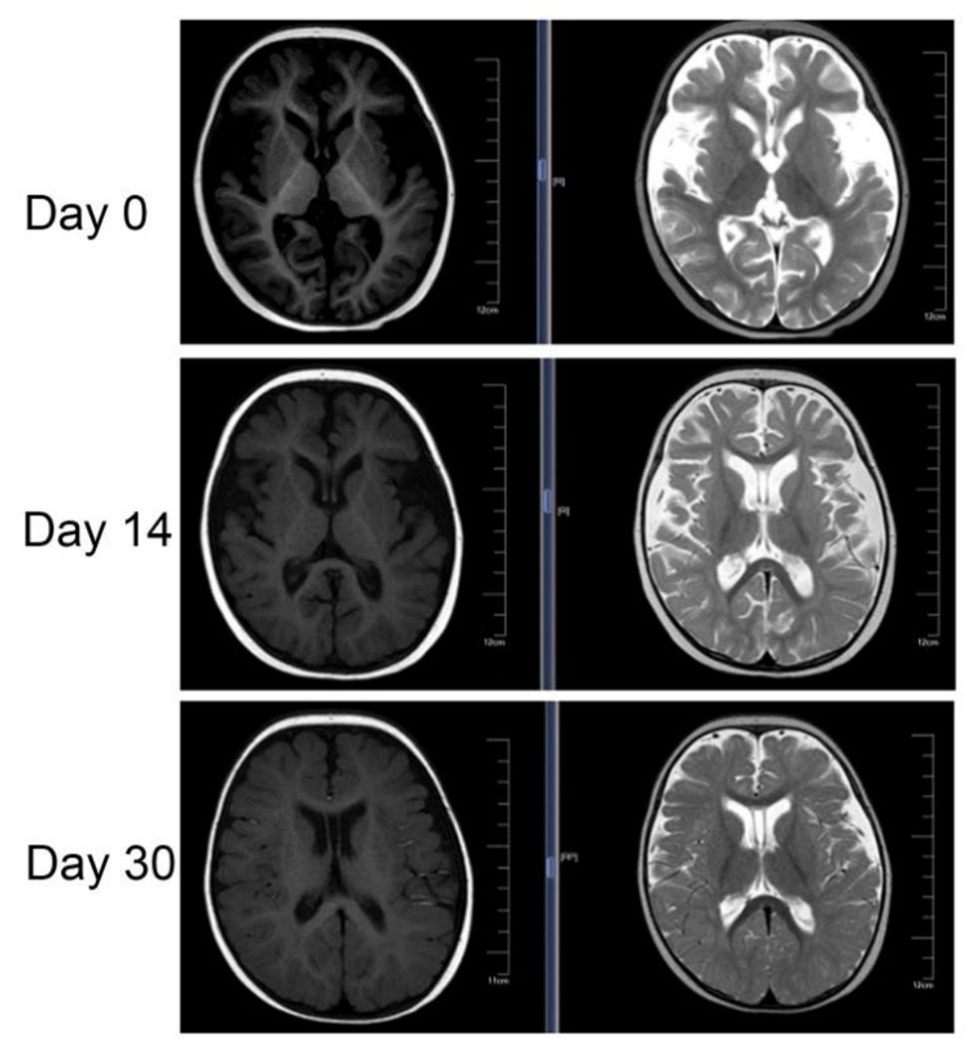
Magnetic resonance imaging showed brain atrophy before and after treatment of the patient
